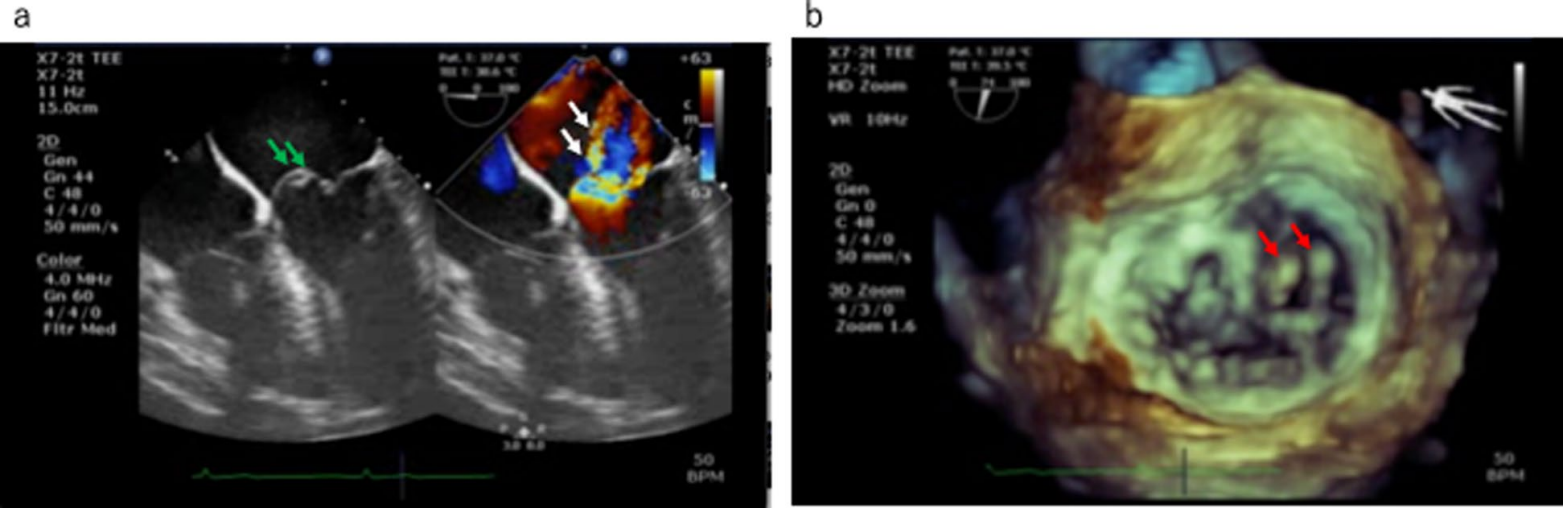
a Mid-esophageal four chamber view and b three-dimensional transesophageal echocardiography in case 1. a Aneurysm-like change (green arrows) in the center of the anterior leaflet (left), with two regurgitation jets apparently in the center of it (white arrows) (right), but with no regurgitation orifices. b Three-dimensional view demonstrates prolapse of the anterior (A3) and of posterior commissure segment (red arrows)
